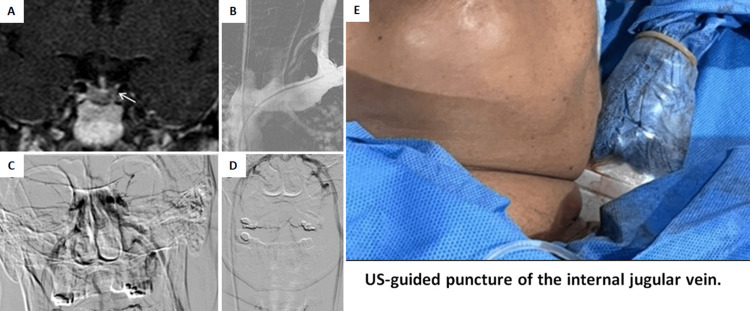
(A) Contrast-enhanced pituitary MRI showing a hypointense nodule in the left half of the gland, which was inconclusive; (B) right internal jugular vein access achieved, while left jugular access was not possible via this route; (C and D) dual inferior petrosal sinus catheterization with right-sided access via the femoral vein and left-sided access via direct jugular puncture; (E) ultrasound-guided placement of the venous sheath.
